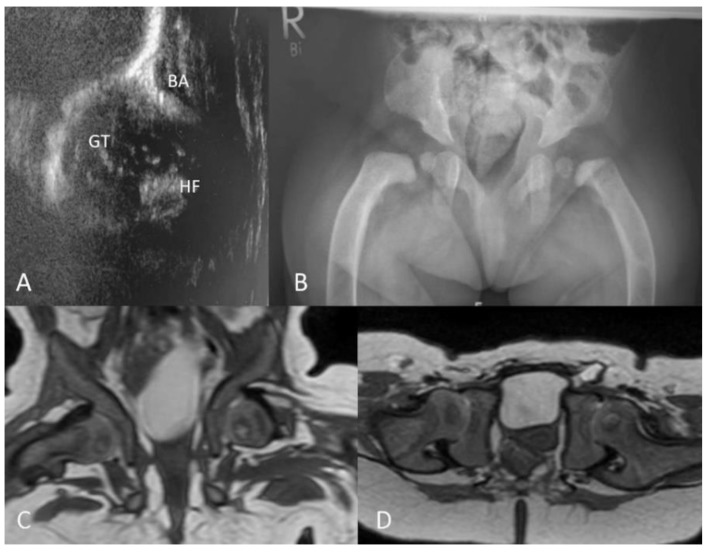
Hip morphology in a female MLII patient (*n* 3). (A) Ultrasound of the right hip at 1 week of age with an overriding greater trochanter; GT, greater trochanter; HF, head of the femur; BA, bony acetabulum. (B) Plain radiograph of the pelvis at 8 months of age presenting flared iliac wings, femoral bowing, an increased acetabular index, but a continuous Shenton’s line. (C,D) Magnetic resonance imaging (MRI) of the pelvis at 8 months of age, T2 weighted, in coronar and transverse views showing centered hip joints.
